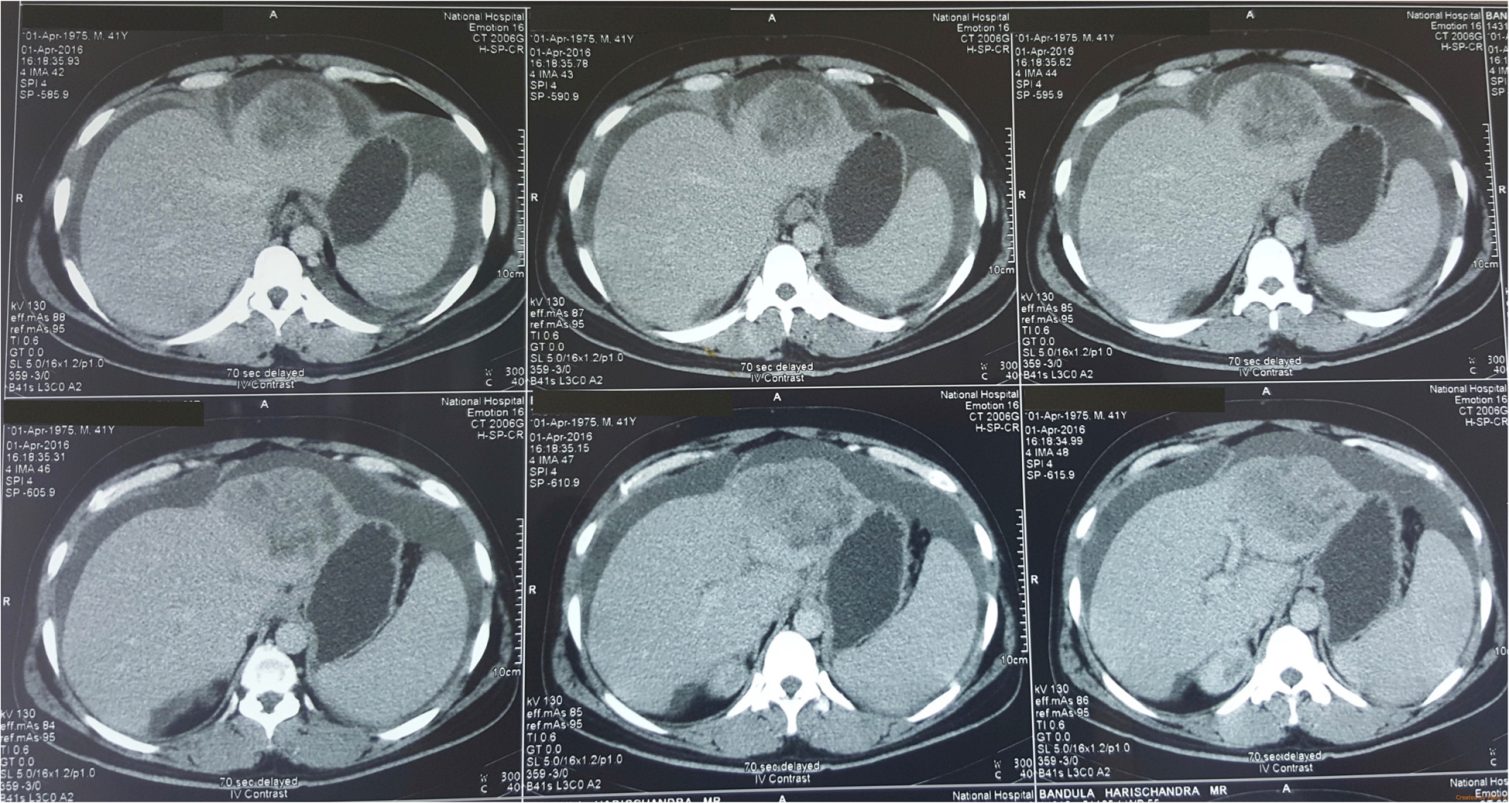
Liver lesion seen in 70 seconds, delayed film. Contrast-enhanced computed tomography of the abdomen
